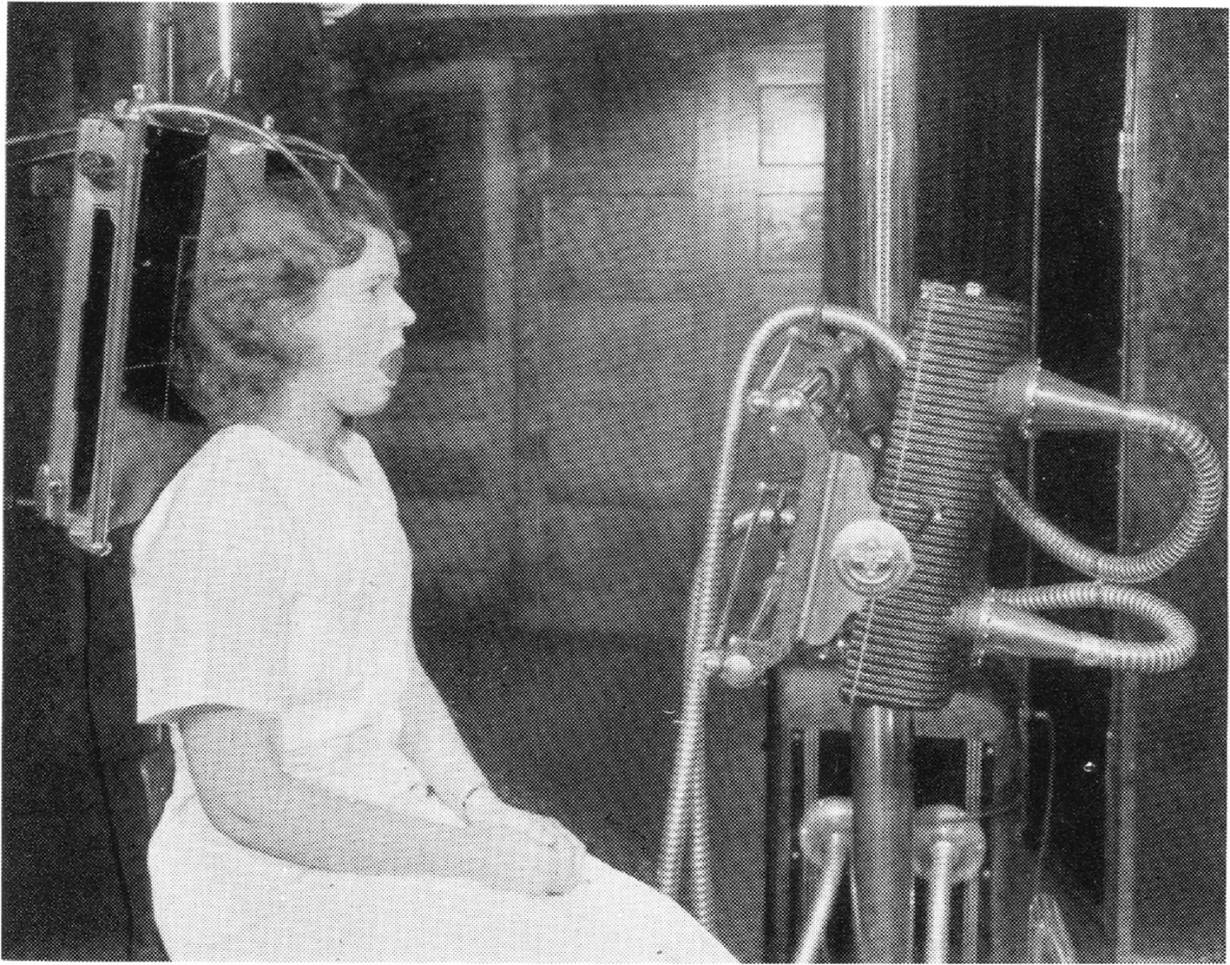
Body positioning devices used in preparation of x-ray for subluxation analysis. (Remier, PA, *Modern X-ray Practice and Chiropractic Spinography*. 1^st^ ed. Davenport, Iowa: Palmer School of Chiropractic, USA, 1938, 260).
